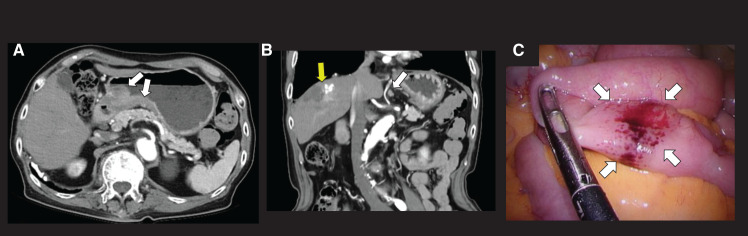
Findings of CT and intraoperative laparoscopic observation. (A) Wall thickening with enhancement from the lesser curvature extending from the gastric angle to the antrum (white arrows). (B) In the coronal section of the CT, enlarged lymph nodes near the gastric cardia to celiac regions (white arrow). Hepatocellular carcinoma after hepatic arterial chemoembolization (yellow arrow). (C) A small intestine tumor was suspected under intraoperative laparoscopic observation.
